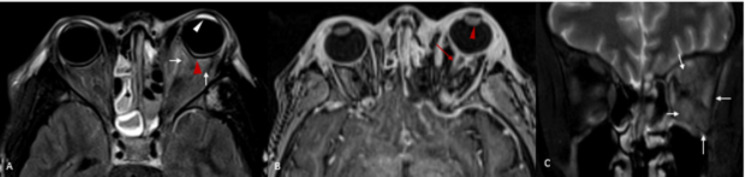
Intra-orbital and optic pathway involvement (A) T2 FLAIR post-gadolinium: diffuse inflammation of left post-septal compartment (white arrows) with left orbital proptosis and tenting of posterior globe (red arrowhead), additional abnormal signal in the left anterior chamber (white arrowhead); B) T1 post-gadolinium MPRAGE: enlarged left lens (red arrowhead) and enhancement and prominence of the left optic nerve sheath (red arrow); C) T2WI coronal: diffuse inflammation of left extra-ocular muscles (white arrows). FLAIR: fluid-attenuated inversion recovery; T2WI: T2 weighted image; MPRAGE: magnetization-prepared rapid acquisition gradient echo
